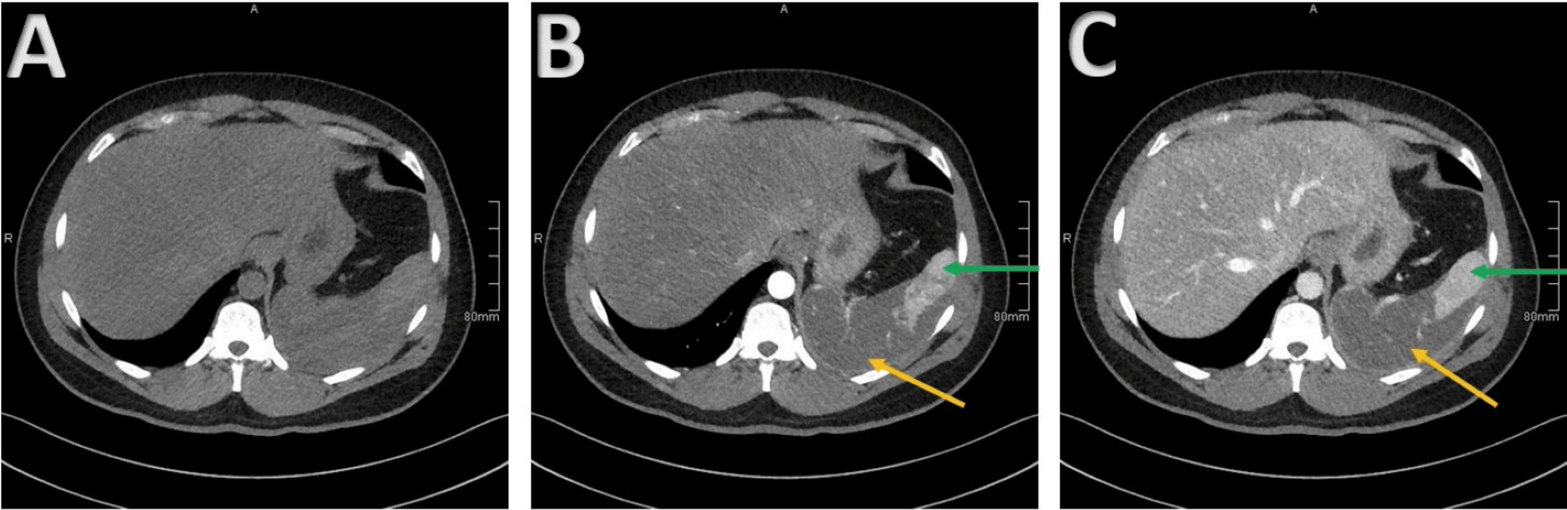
Computed tomography images obtained 10 days after symptom onset Axial non-enhanced (A) and contrast enhanced images in arterial phase (B) and portal venous phase (C) demonstrate a large non-enhancing area of the spleen (orange arrows) consistent with splenic infarction. Normal enhancing spleen is shown anteriorly (green arrows).
